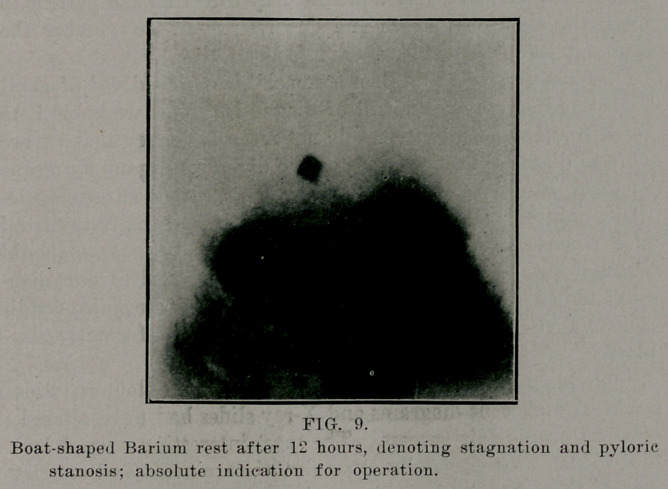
# The X-Ray in Diagnosis of Diseases of the Stomach—With Lantern Slide Demonstration
